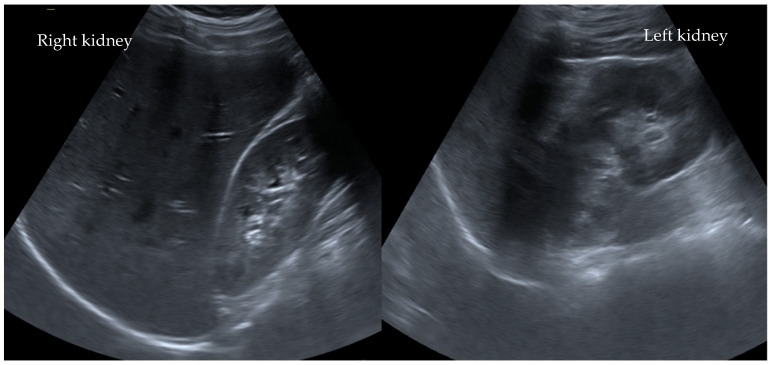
No parenchymal pathologies observed ultrasonographically in the kidneys after ibuprofen misuse in Case I°. Ultrasonographically, there are no parenchymal changes, medullary pyramids, especially sonomorphologically laterally symmetrical and inconspicuous with good corticomedullary parenchymal differentiation. The kidneys are normal in size. The resistance index is laterally symmetrical and normal for age.
